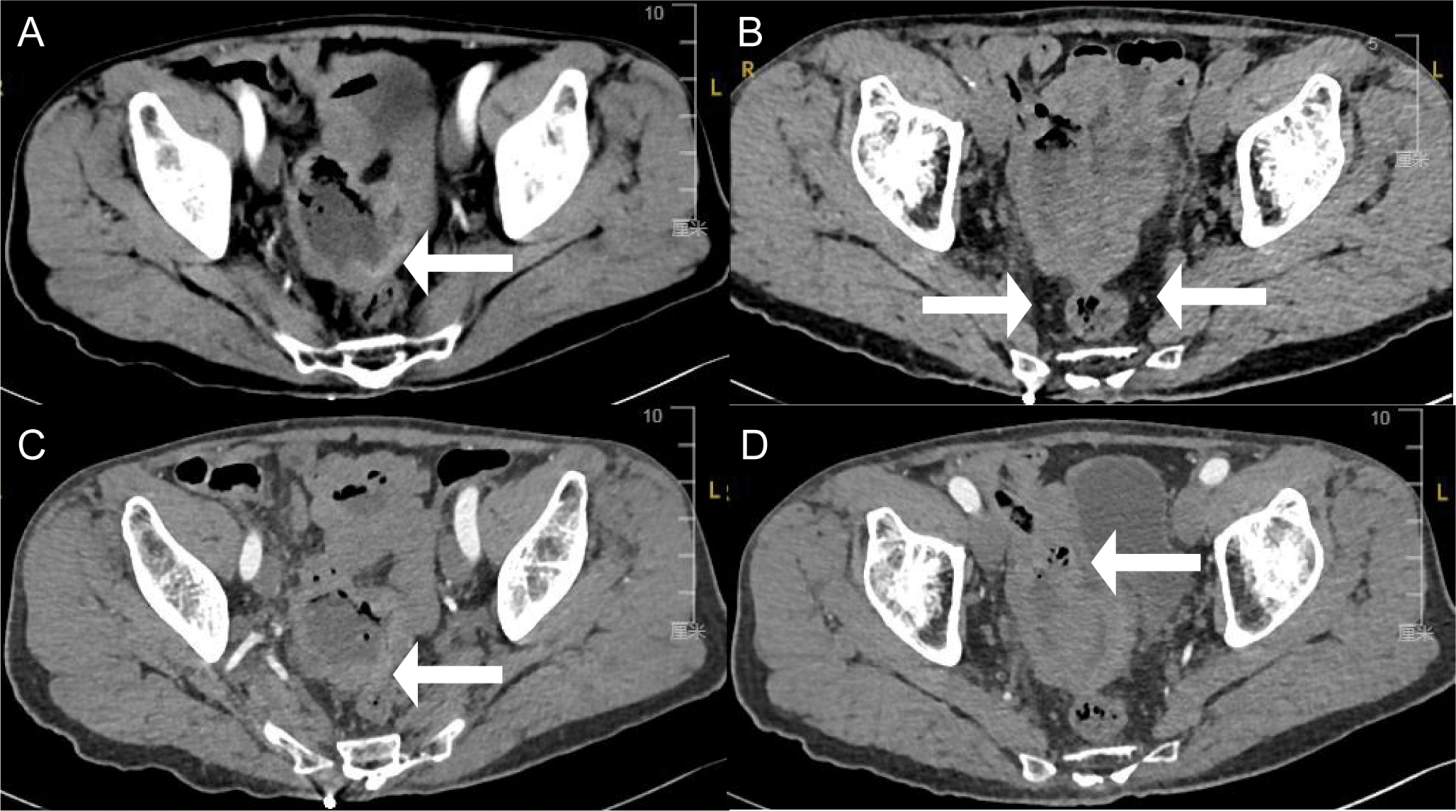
Enhance abdominal computed tomography cross-section images showing (A) thickening of part of the ileal wall (white arrows), (B) multiple small lymph nodes around the lesion (white arrows), (C) indistinct surrounding spaces with the sigmoid colon (white arrows), and (D) closely to the right posterior bladder wall (white arrows).
